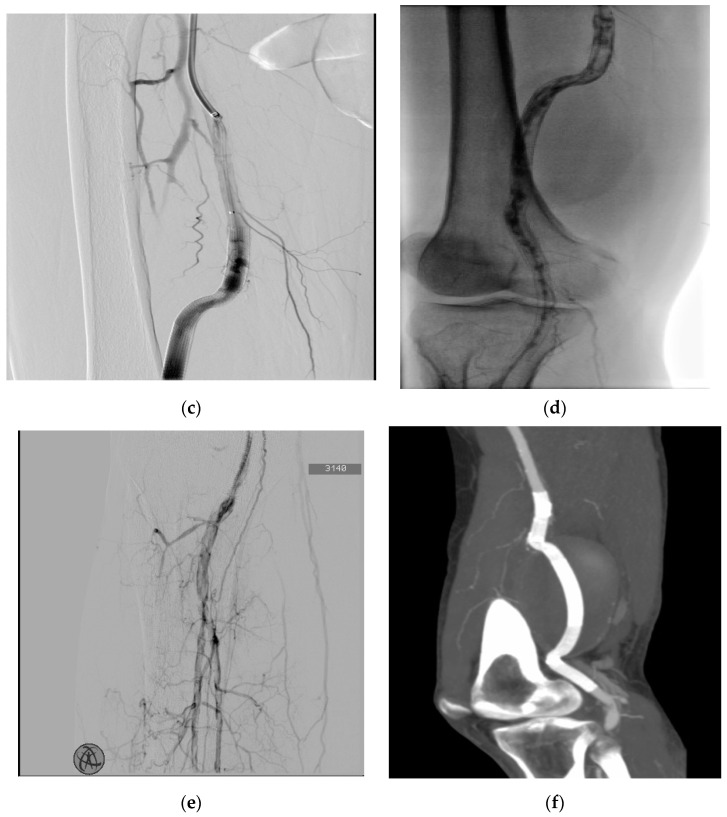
(a) Preoperative CTA, showing the ruptured PAA in the lateral projection and a focal rupture in the rectus femoris muscle in the axial projection in the yellow box marked by narrowing. (b) Final intraoperative radiographic control showing the VIABAHN™ endoprosthesis and Supera stent in the popliteal artery after the first endovascular emergency procedure. (c) An intraoperative angiography through the catheter shows proximal and (d) distal intrastent thrombosis. (e) Intraoperative complication with thrombosis of the anterior tibial artery and peroneal trunk. (f) Computer tomography angiography shows a perianeurysmal collection of approximately 9 cm and endovascular bypass patency.
